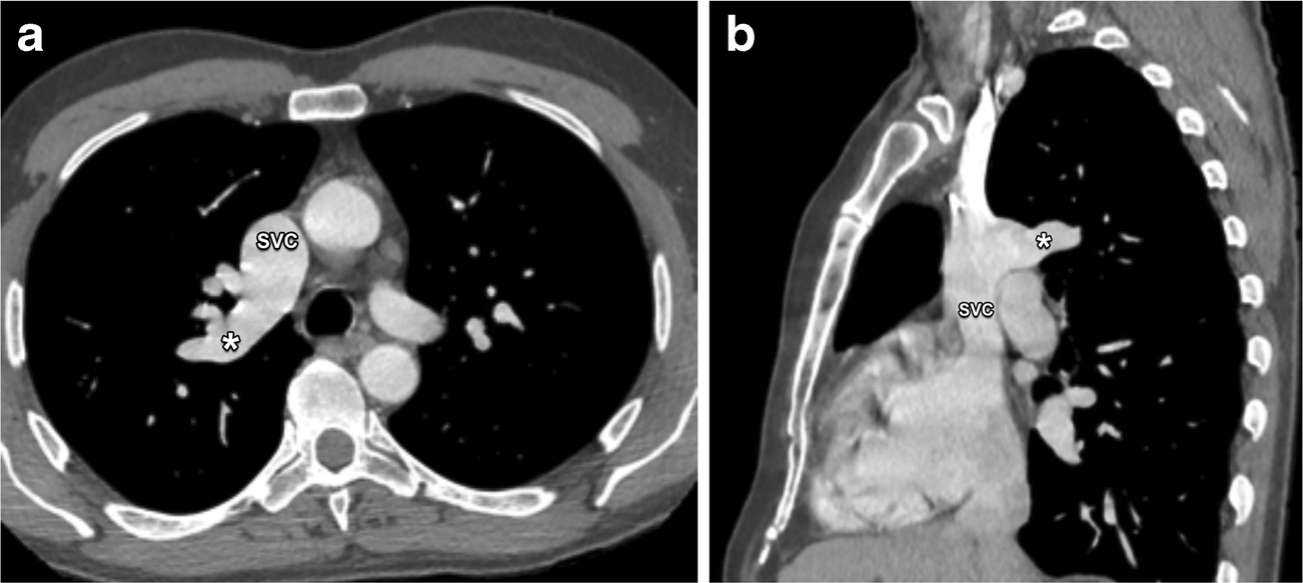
Axial (a) and oblique sagittal (b) post-contrast CT images of a patient with anomalous return of the right upper pulmonary vein (*asterisk*) in the superior vena cava (SVC)
